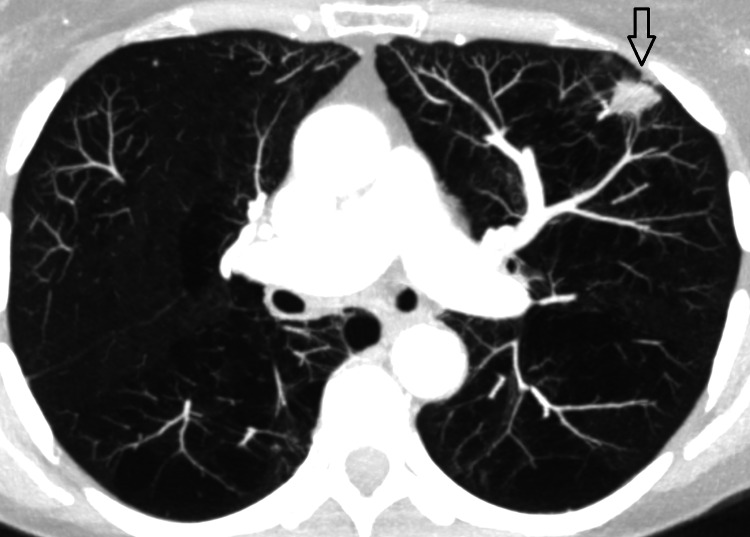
Axial CT scan showing the largest of five lung metastases (anterior part of the left lung, indicated by arrow). CT, computed tomography
